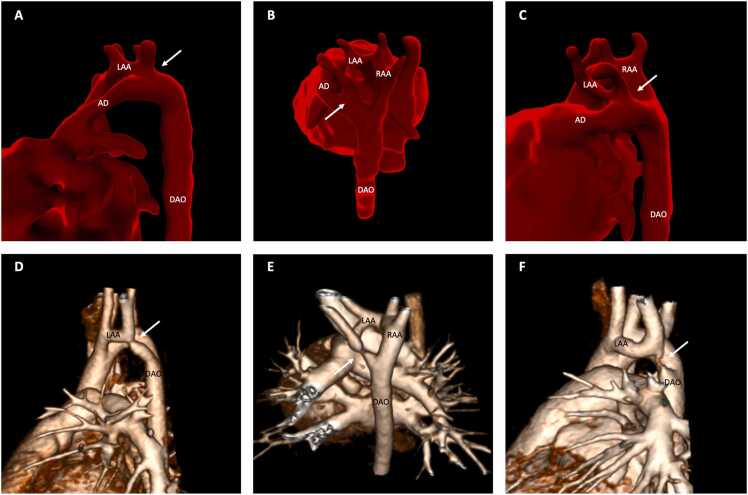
DAA with asymmetrical arches and postnatal coarctation of smaller arch. Third-trimester fetal CMR (A-C) showing the LAA coursing posterior to the trachea but with a degree of anterior displacement of the left aortic isthmus (arrowed) before joining the left-sided arterial duct/descending aorta. Corresponding contrast-enhanced CT demonstrated discrete coarctation (D and E) or severe hypoplasia (F) with luminal continuity of the left aortic isthmus (arrowed). *AD* arterial duct, *DAO* descending aorta, *RAA* right aortic arch, *LAA* left aortic arch, *DAA* double aortic arch, *CMR* cardiovasuclar magnetic resonance, *CT* computed tomography.
